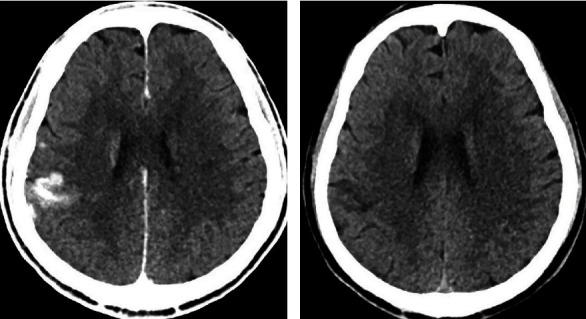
Non-enhanced brain CT scan of a 58-year-old woman who had a sudden onset of impaired left limb movement. (a). The non-enhanced brain CT scan obtained immediately after intra-arterial thrombolysis showed that a type I hyperdense lesion was located in the right temporal lobe cortex with a CT value of 90-135 Hu. There was no mass effect. (b). Follow-up CT scan obtained 24 hours after the end of AR, the hyperdense lesion on the right temporal lobe cortex was completely disappeared.
